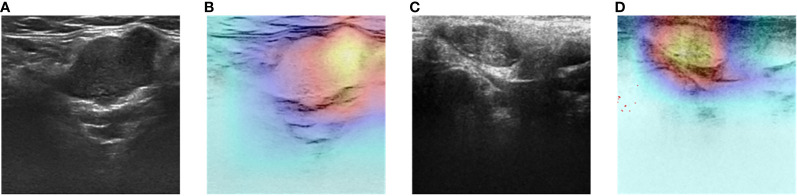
Two-dimensional ultrasound images and heatmaps of parotid gland tumor: (A) ultrasound image of PA; (B) heatmap of the same PA; (C) ultrasound image of WT; (D) heatmap of the same WT.
